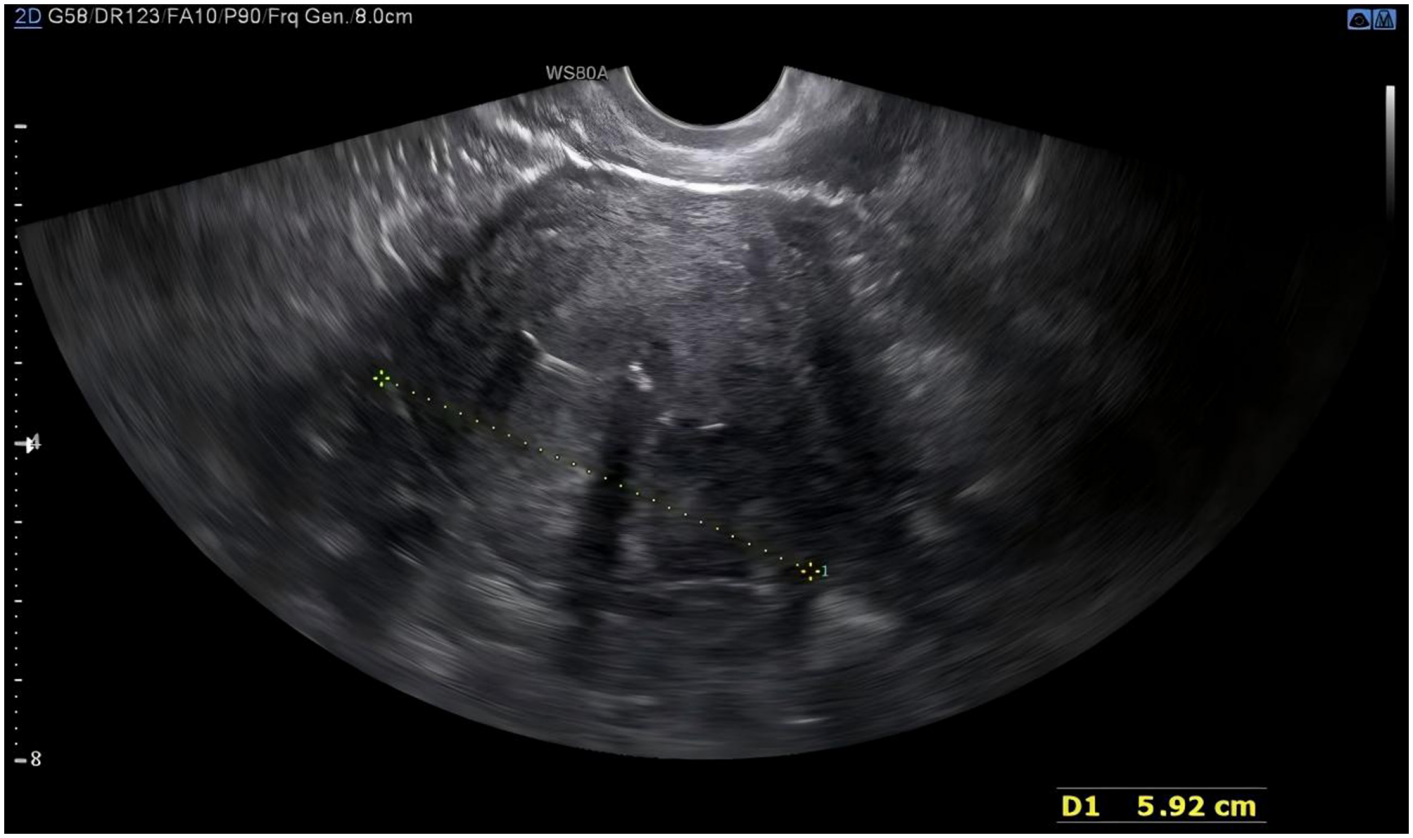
Post-operative vaginal ultrasound showed that the LNG-IUS was in a normal position within the uterine cavity.
